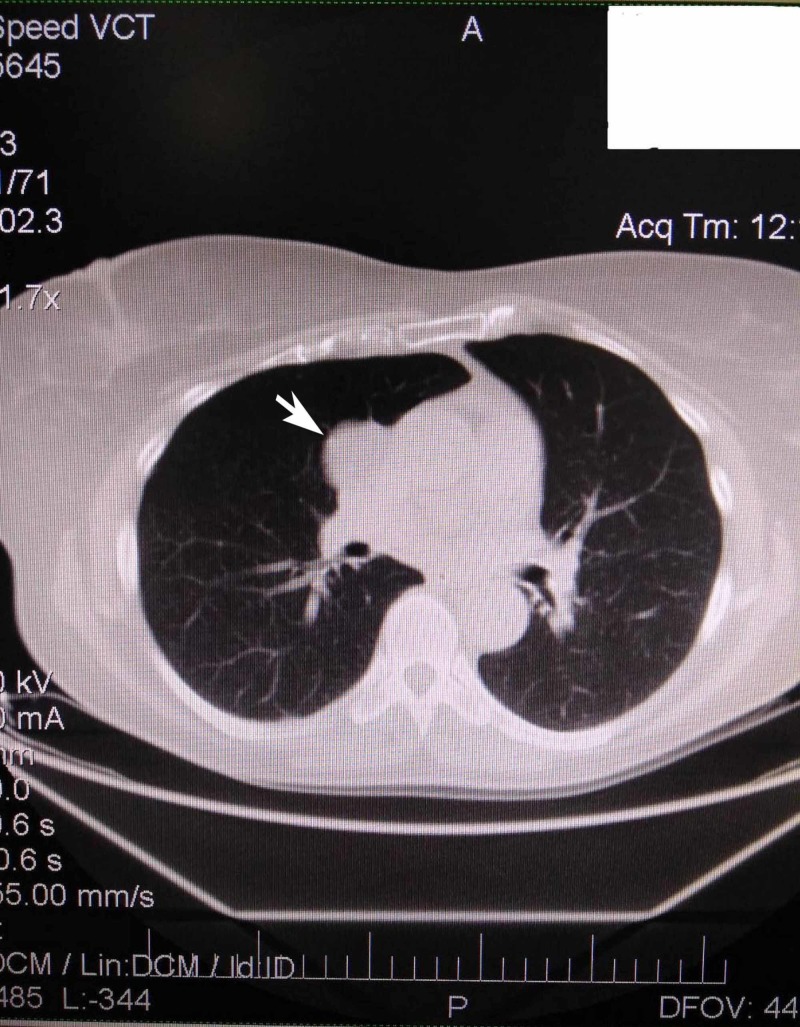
Enhanced CT-scan with a solitary lesion in the right side medial mediastinum
